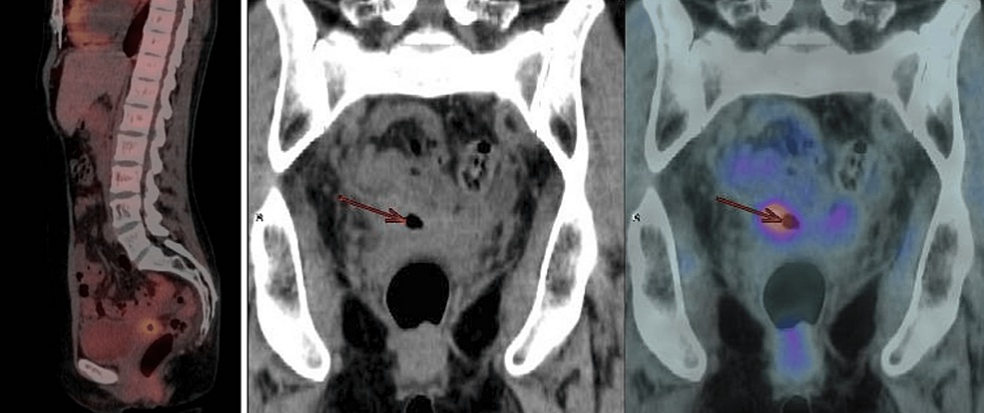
Positron emission tomography associated with computerized tomography demonstrated increased metabolic expression in the vaginal vault (SUVmax: 4.5 / late: 4.6). There was moderate dilatation of the bilateral ureters.
